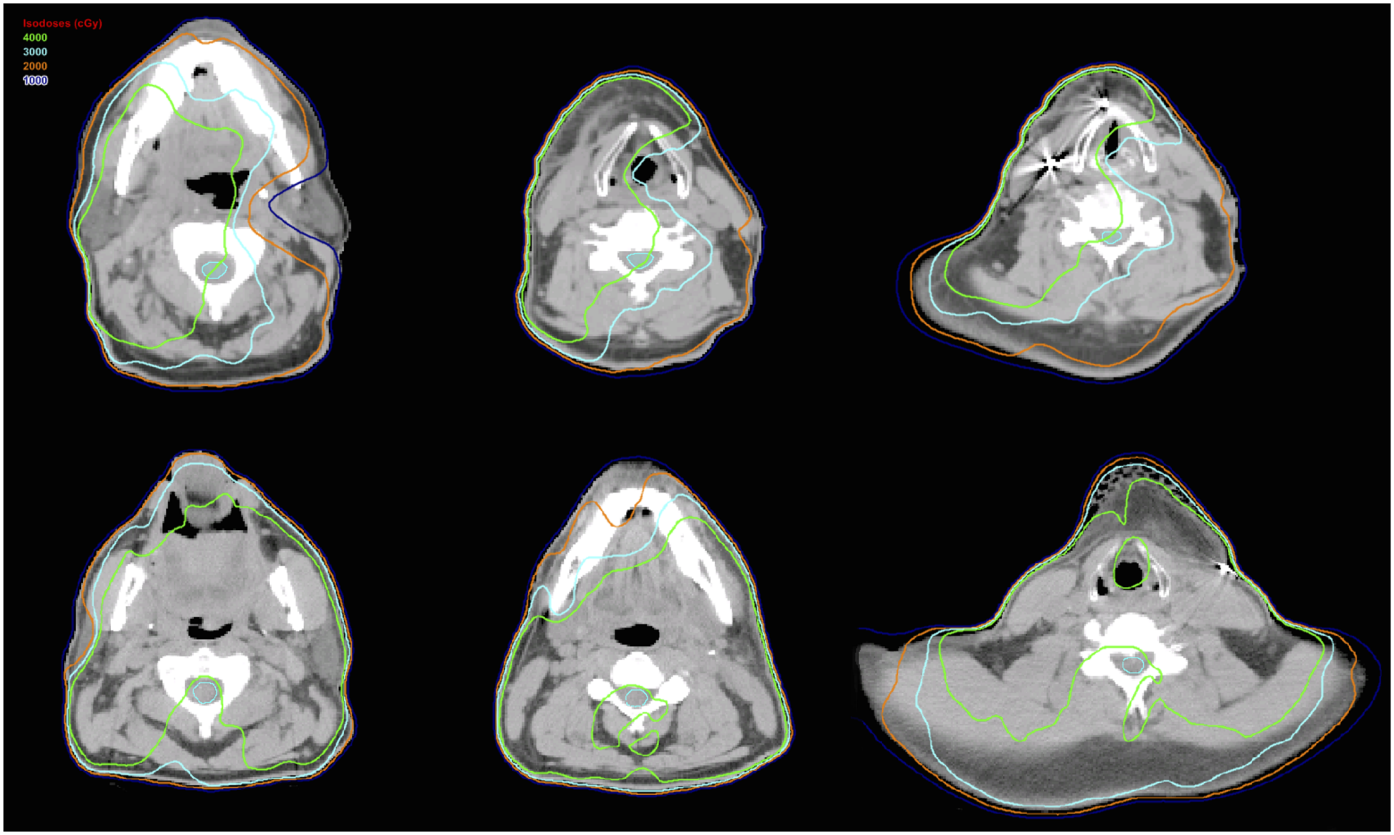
Axial CT images of an LS patient, treated unilaterally (top row), and a non-LS patient, treated bilaterally (bottom row). Note that in the unilaterally treated patient, the 40 Gy line (green) hemisects the spinal cord.
